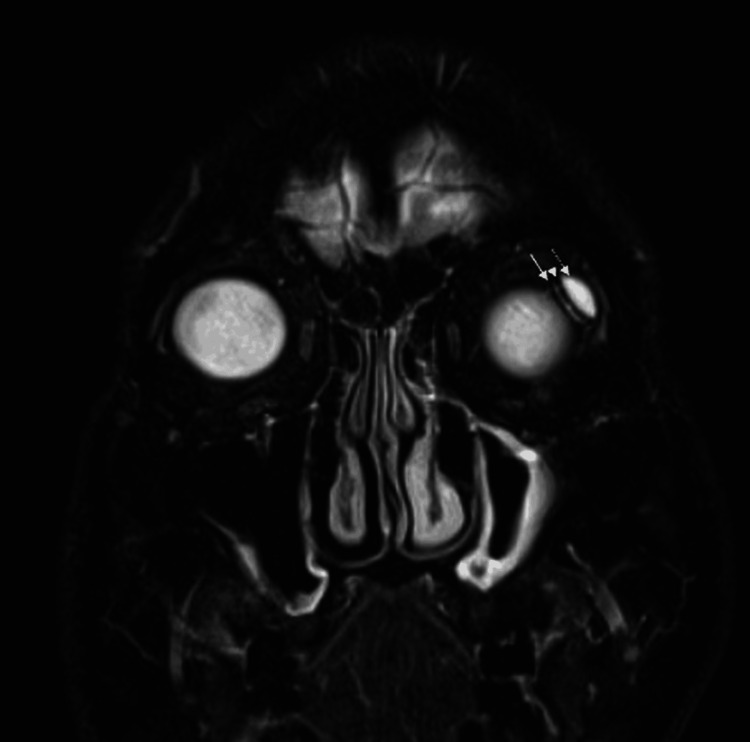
Orbital MRI after upside-down pars plana Ahmed glaucoma valve implantation The operated eye is shown on the right side of the image. A representative T2-weighted coronal orbital MRI obtained at approximately six years postoperatively demonstrates a hyperintense fluid signal consistent with a peri-endplate bleb. A double-layered bleb is observed on the scleral side (solid arrow) and conjunctival side (dashed arrow) of the endplate. The endplate is identified as a low-intensity structure between the bilayered bleb (arrowheads) MRI: magnetic resonance imaging
